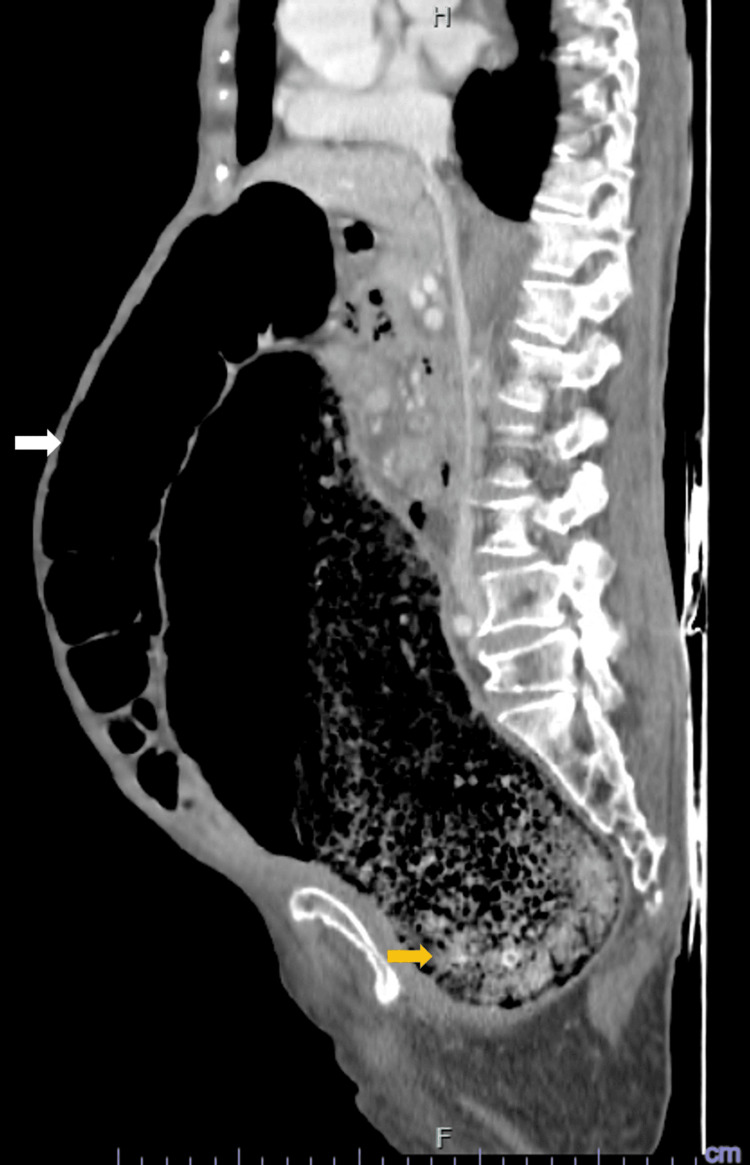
Sagittal CT findings in acute colonic pseudo-obstruction. Sagittal computed tomography (CT) image demonstrating marked colonic dilation (white arrow) with a large rectal fecaloma (yellow arrow), consistent with acute colonic pseudo-obstruction (Ogilvie syndrome).
